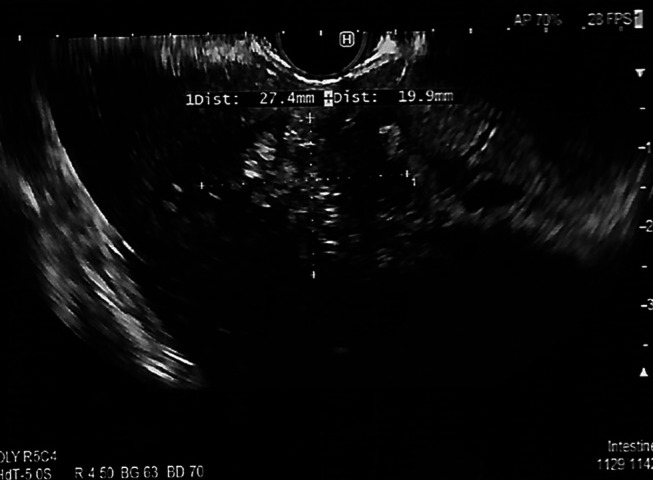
Endoscopic ultrasound of the liver mass.
